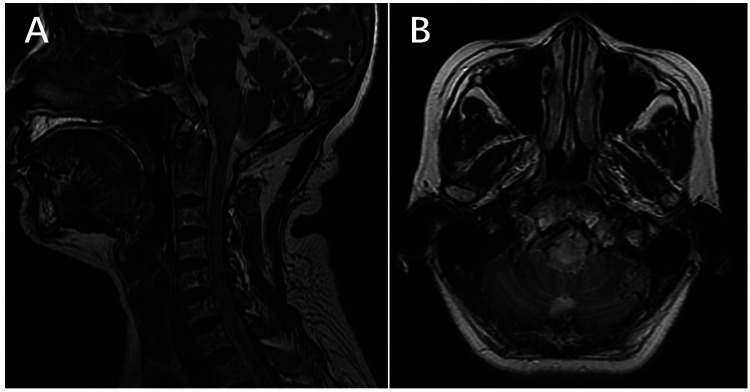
Preoperative magnetic resonance imaging (MRI). (A) and (B): MRI showing extensive edema of pons with flow voids over its surface.
